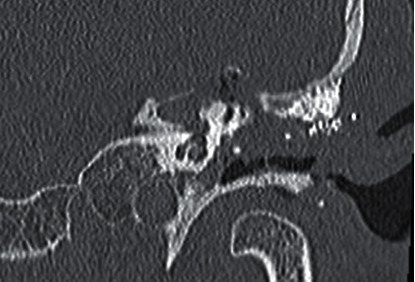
Coronal CT of the amputated superior semicircular canal (asterix) and the postoperative defect of the left petrous apex.
